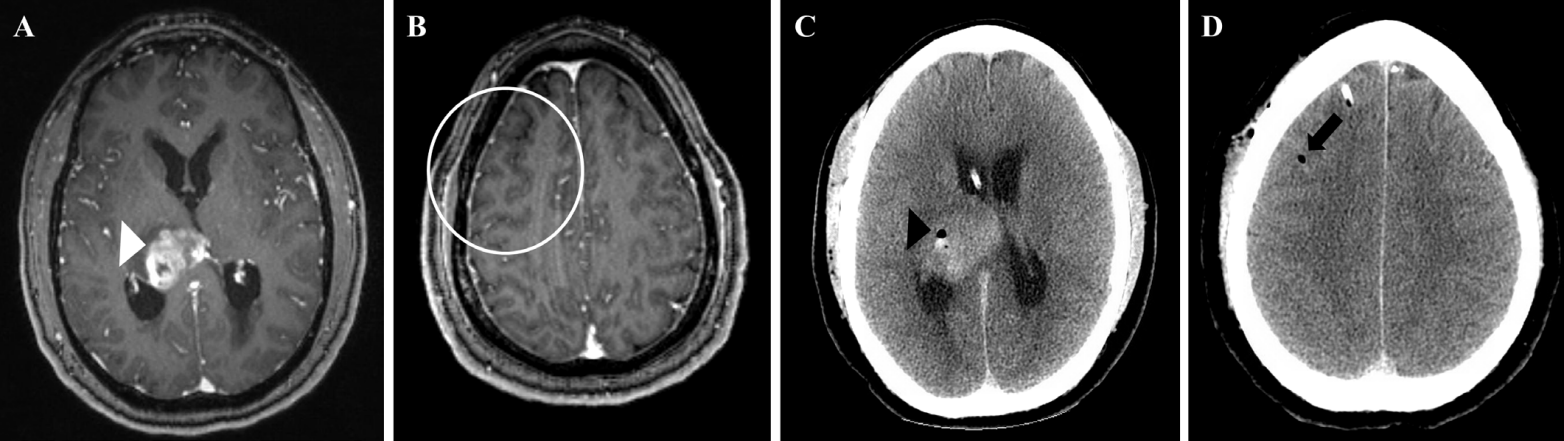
Imaging at the time of tumor diagnosis. Axial contrast-enhanced T1WMRI showing the primary lesion (white arrowhead), (A) and no secondary lesion in the right middle frontal gyrus (white circle), (B) at the time of diagnosis. Axial CT taken after patient underwent stereotactic biopsy of the primary lesion. Air in the tissue sampling location within the lesion (black arrowhead), (C). Higher level slice demonstrating air in the biopsy tract in the future site of the metastatic lesion (white arrow), (D).
